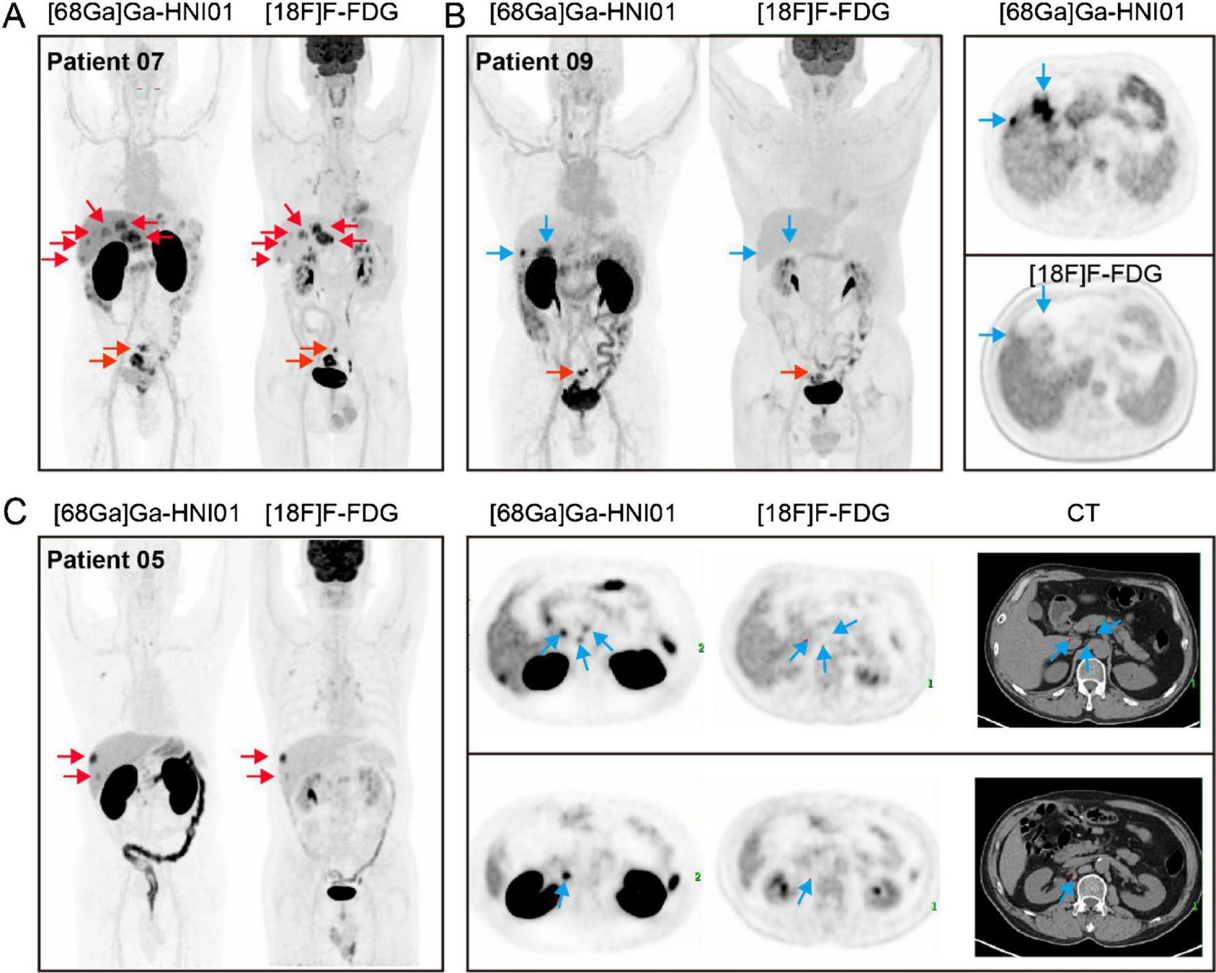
Comparative PET imaging of a CEA-targeting nanobody, [^68^Ga]Ga-HNI01, and [^18^F]F-FDG in colorectal cancer metastases. A) MIP images from [^68^Ga]Ga-HNI01 PET/CT (left, at 2 h p.i.) and [^18^F]FDG PET/CT (right) in a patient with liver metastases and primary lesions. [^68^Ga]Ga-HNI01 PET/CT and [^18^F]FDG PET/CT both detected the primary and hepatic lesions (red arrow), indicating the ability of [^68^Ga]Ga-HNI01 to detect liver metastasis of colorectal cancer (CRC). B) The MIP (left) and transaxial (right) PET images in a second patient showed that the liver metastases (blue arrow) exhibited only high uptakes of [^68^Ga]Ga-HNI01, whereas [^18^F]FDG was negative. The primary lesions (red arrow) of patient 09 were detected by both tracers. C) The MIP (left) and transaxial (right) PET images in a third patient. The MIP images showed that hepatic lesions (red arrow) were clearly detected by both tracers. However, the lymph node metastases (blue arrow) in the liver were seen only by [^68^Ga]Ga-HNI01 and showed low [^18^F]FDG accumulation. This image was adapted from Li L, Lin X, Wang L, et al. Immuno-PET of colorectal cancer with a CEA-targeted [^68^Ga]Ga-nanobody: from bench to bedside. Eur J Nucl Med Mol Imaging. 2023;50(13):3735–49
